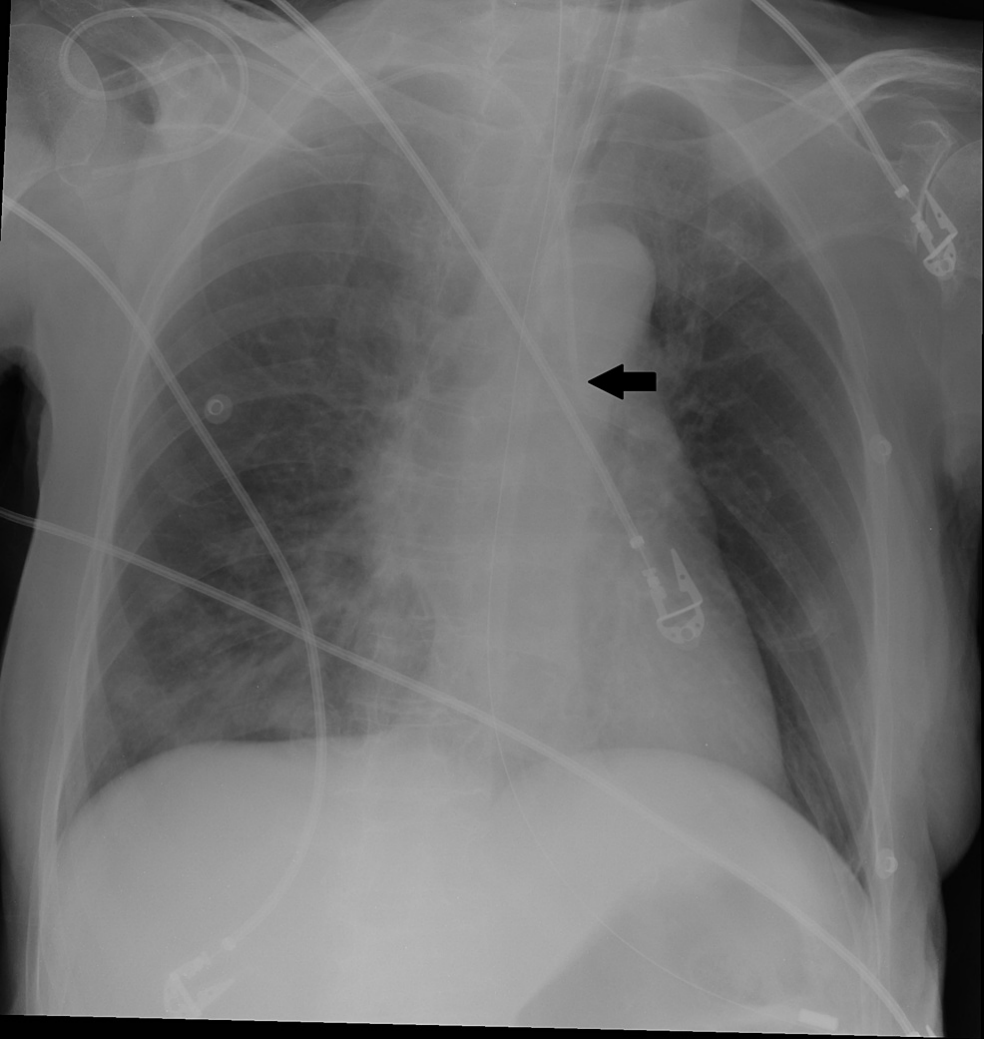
Chest X-ray confirming the placement of the central line
